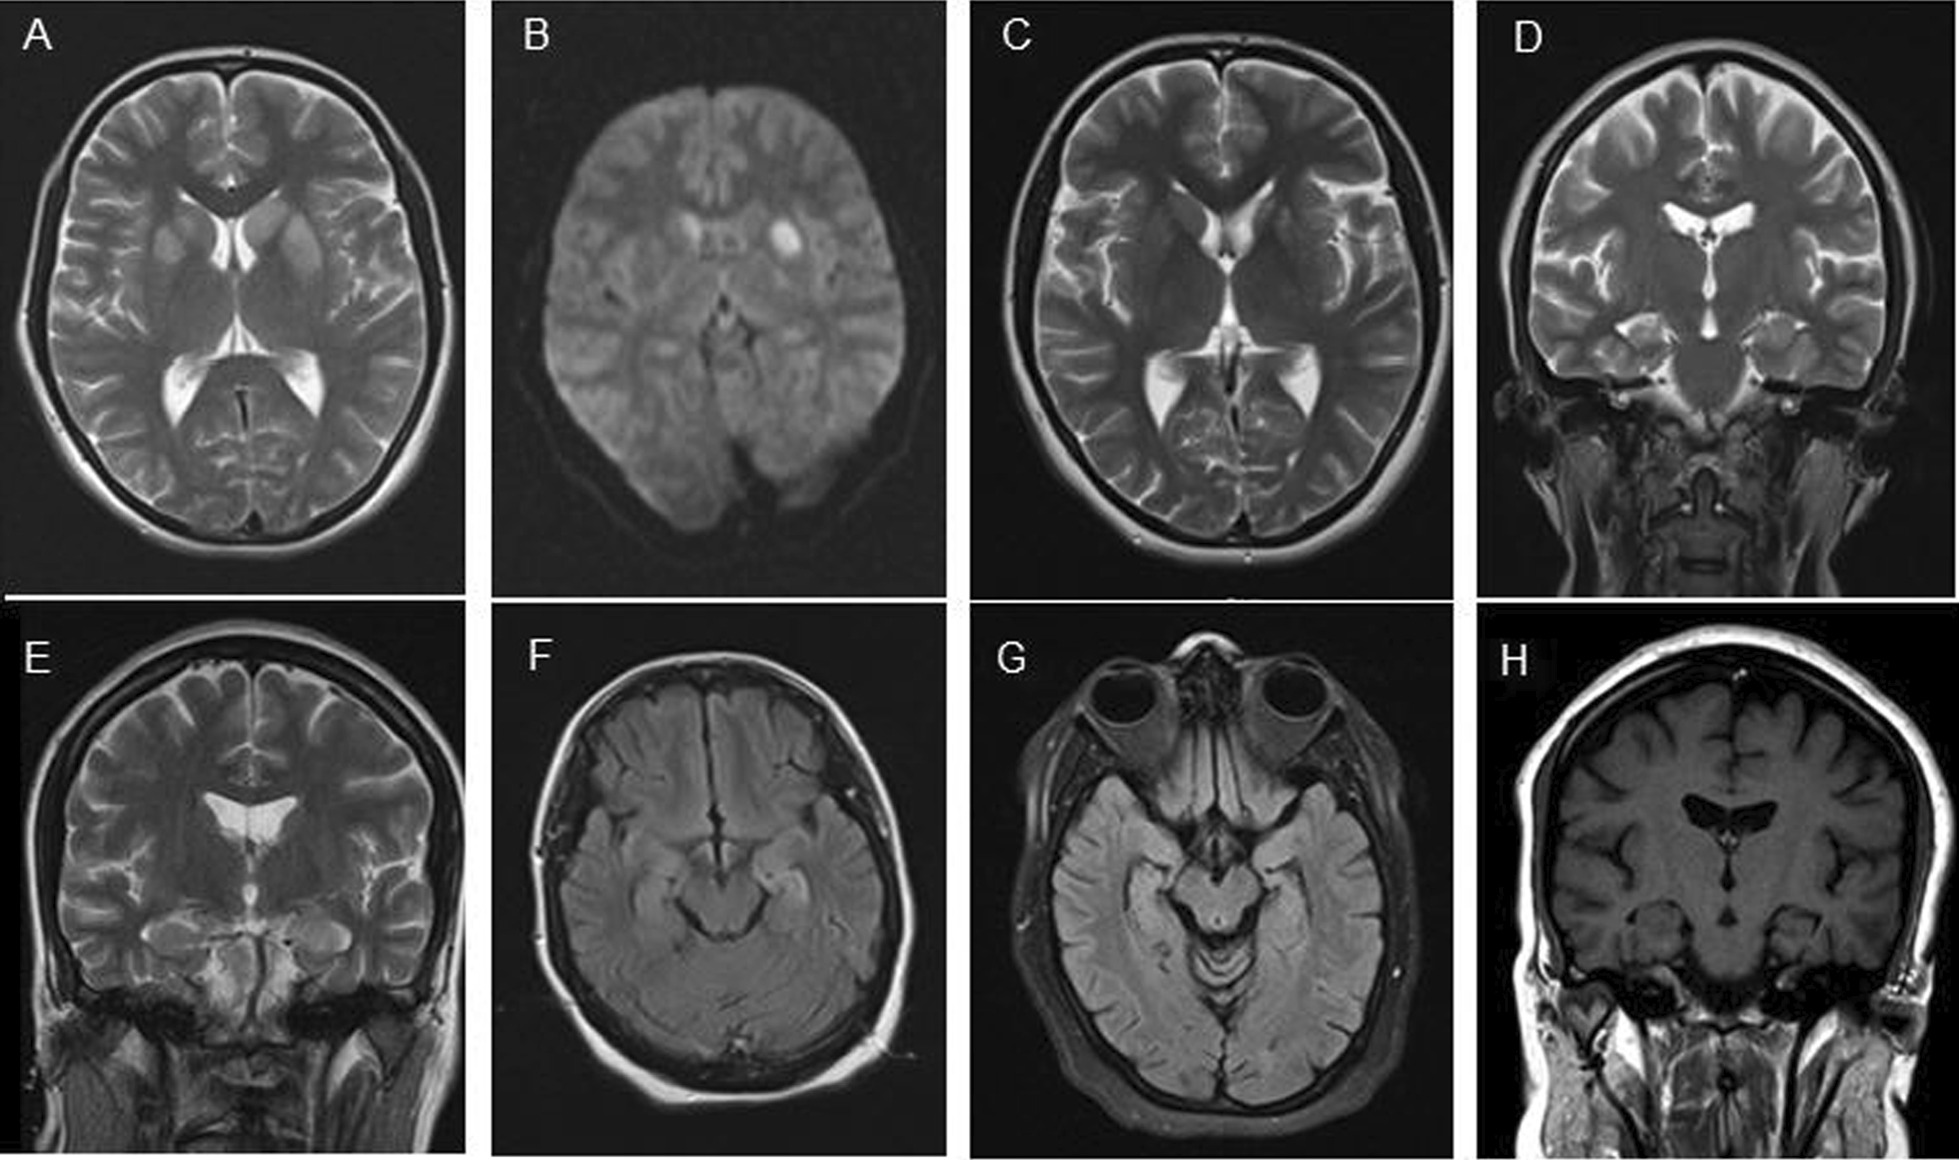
Case 1: bilateral hyperintense lesions in basal ganglia (left more than right) in T2 sequence (A). Left basal ganglia restriction in diffusion-weighted imaging (B). Significant decrease in Magnetic resonance imaging hyperintensities in the basal ganglia on 6-month follow up Magnetic resonance imaging (C) and right hippocampal sclerosis (D). Case 2: Transvere relaxation time 2- Fluid-attenuated inversion recovery hyperintensities in the left mesiotemporal structures (E, F). Mild left-side hippocampal sclerosis on the 1-year follow-up Magnetic resonance imaging (G, H)
